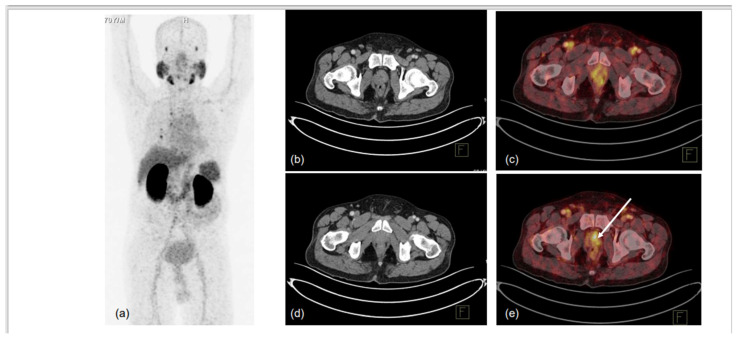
70-year-old male, presented with difficulty in urination and lower urinary tract symptoms (LUTS). Serum PSA was 0.43 ng/mL. mpMRI of prostate was performed, which showed indeterminate lesion in right peripheral zone of mid-gland region. ^68^Ga-PSMA-11 PET-CT was used to localise prostate lesion for targeted biopsy. MIP (a) showed low-grade PSMA uptake in prostate, with non-specific uptake in right lung and normal biodistribution. CT trans-axial slices and corresponding fused PET-CT trans-axial slices show mildly PSMA-avid hypodense lesion in right peripheral and central zones in mid-gland region (1.0 × 1.0 cm, SUVmax 5.6) (b–e). No locoregional or distant metastases were noted. TRUS-guided biopsy of PSMA-expressing lesion showed infiltrating acinar adenocarcinoma, Gleason grade 3 + 3/10. Arrow in ‘e’ denotes the PSMA-expressing area submitted for biopsy.
